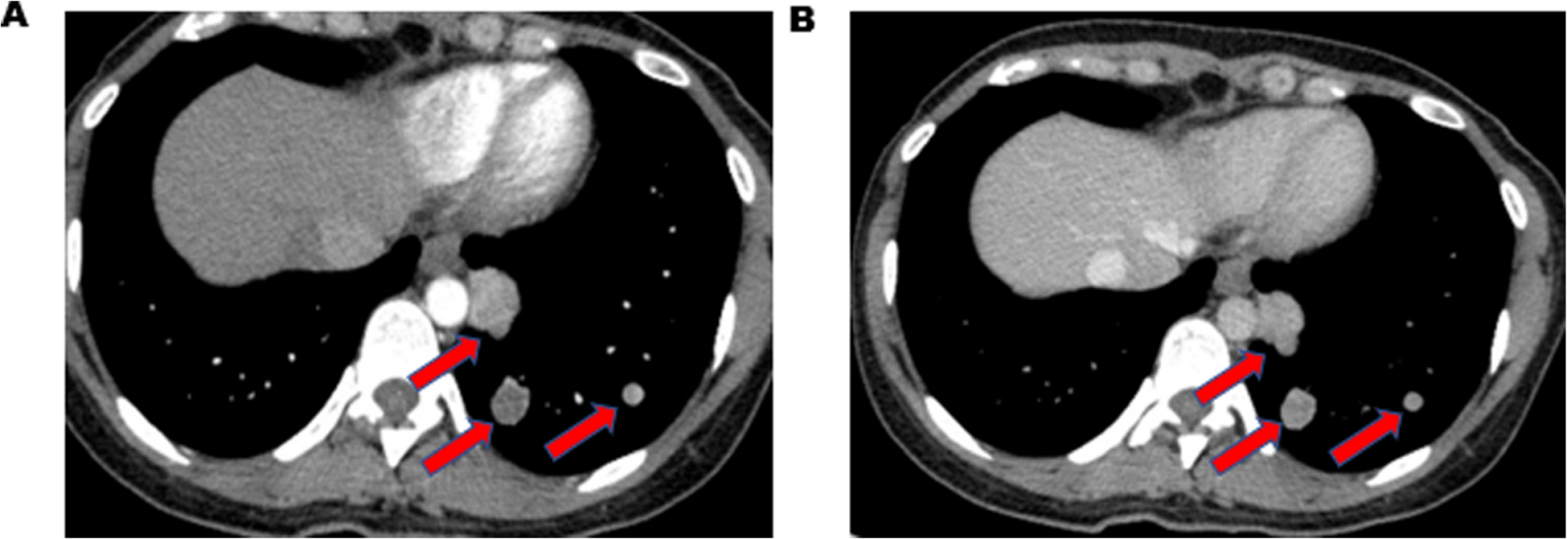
a chest CT revealed intense heterogeneous enhancement in the aterial phase. b chest CT showed nodules’ homogeneous nature in the delay phase
